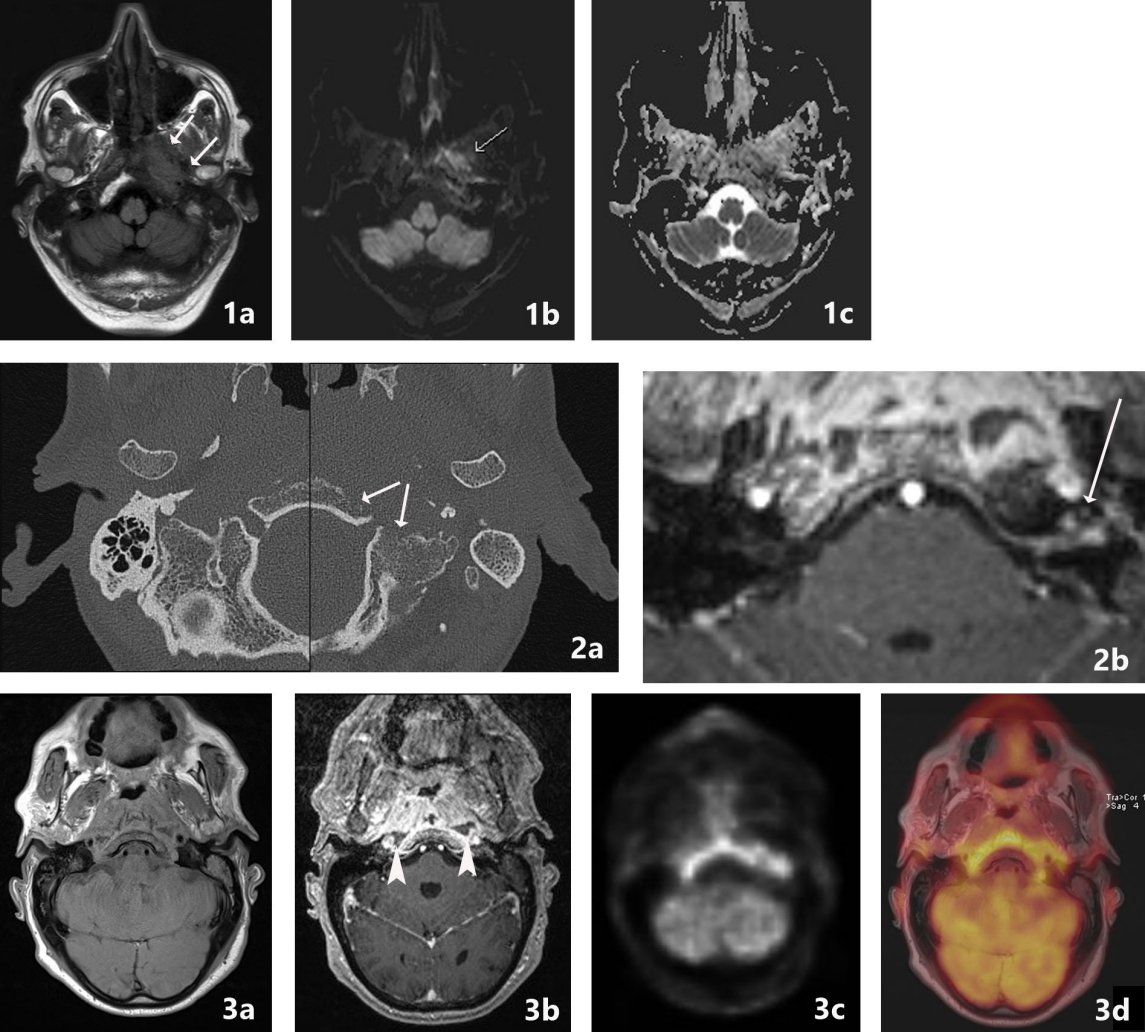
Abscess formation and involvement of facial nerve. A 65-year-old male with a history of a kidney transplant presented with headache and cranial nerve deficit, with dysphonia and facial nerve palsy. Otoscopy revealed a red and painful EAC. At MRI, there is SBO involvement of the bone marrow of the temporal bone and the clivus, with medial spreading pattern of the soft tissues (image 1a arrows). There is a hypointensity of the subtemporal soft tissues with obliteration of normal fatplanes in the masticator space. At DWI, a slight hyperintensity is shown (image 1b arrow), with relative low ADC (image 1c). At high-resolution CT demineralization and cortical destruction of the skull base and clivus can be appreciated (image 2a arrows). Follow-up PET-MRI (image 3a T1-w, 3b CE-T1-w) 1 month later shows SBO with expansion over the midline (crossed pattern) and abscess formation bilateral at the prevertebral region (image 3b arrowheads), with intense FDG avidity (image 3c PET, 3d colour fused PET-MR image). Involvement of the VII cranial nerve was seen at contrast-enhanced T1-w imaging, without osseous destruction of the facial canal (image 2b detail of skull base on T1-w-fs, arrow indicates enhancing facial nerve) (Color figure online)
